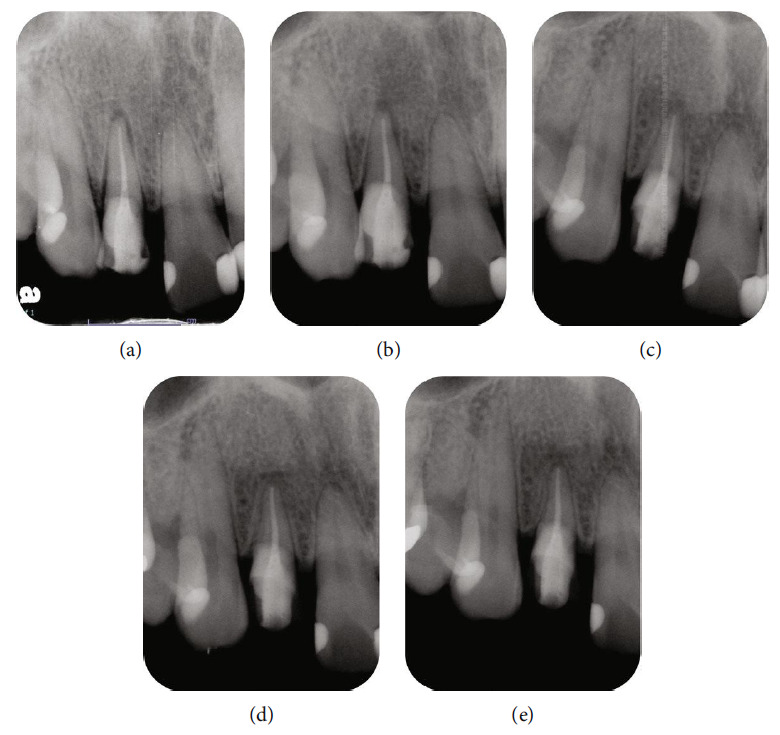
Radiographic images after intentional replantation. (a) Intraoral radiographic image was taken immediately after intentional replantation with crown rotation. (b) Intraoral radiographic image was taken one month after intentional replantation. The periodontal ligament space appears to be slightly larger than that immediately after intentional replantation (c) Intraoral radiographic image was taken three months after intentional replantation. The periodontal ligament space is similar to that around the adjacent teeth. (d and e) Intraoral radiographic images were taken six months and one year after intentional replantation, respectively. Low-contrast component resin crowns are bonded with adhesive resin.
